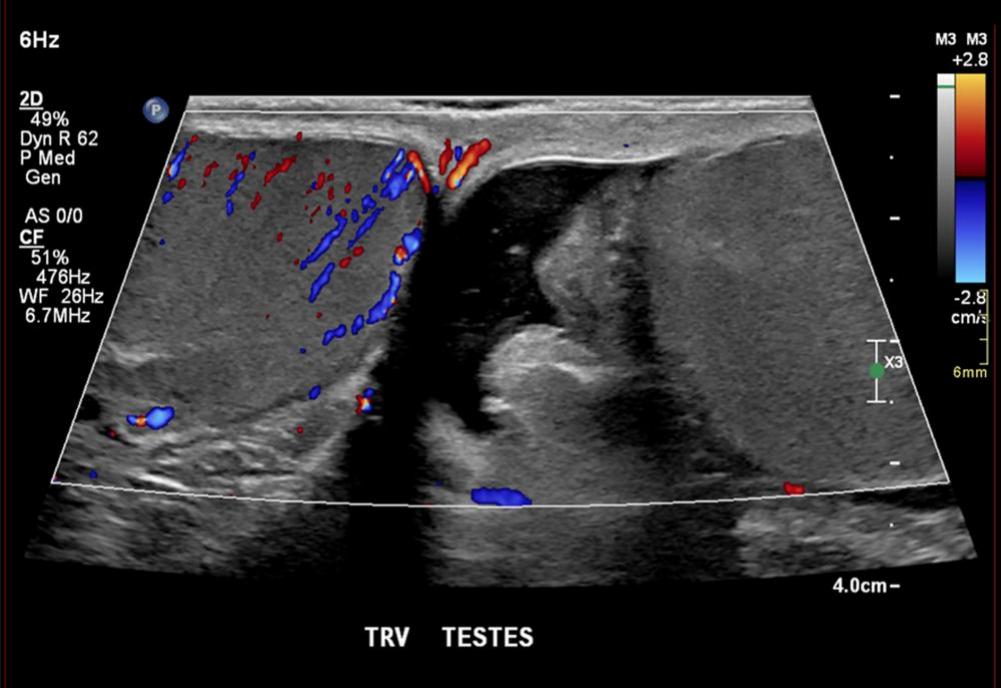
Left-sided testicular torsion. Doppler interrogation demonstrates a complete lack of blood flow in the mildly enlarged, edematous left testis (right side of the image) relative to the normal contralateral side.
